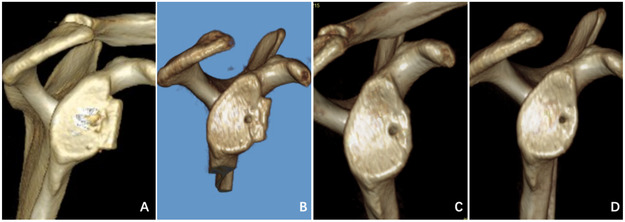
Right side. Serial postoperative CT scans (immediate (A), 3 months (B), 6 months (C), and 1 year (D)) show graft incorporation, restoration of glenoid contour, and bone resorption occurring outside the glenoid best‐fit circle. (CT, computed tomography.)
